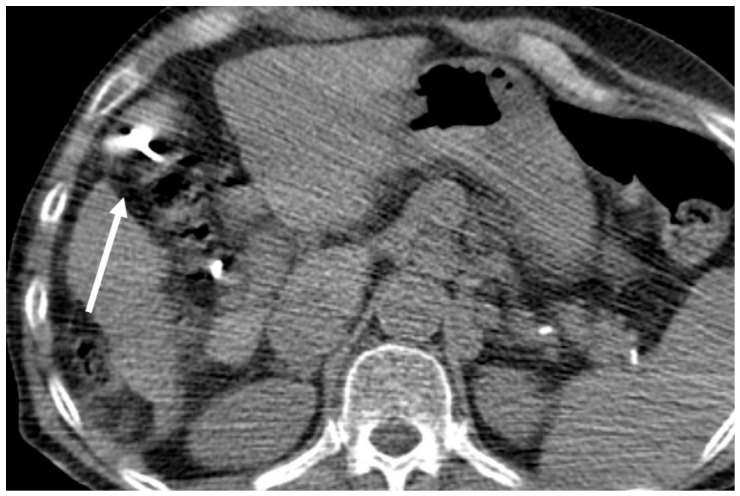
Right: Axial CT scan shows the accidental transgression/close proximity of the ablation needle through the bowel loops (arrow).
